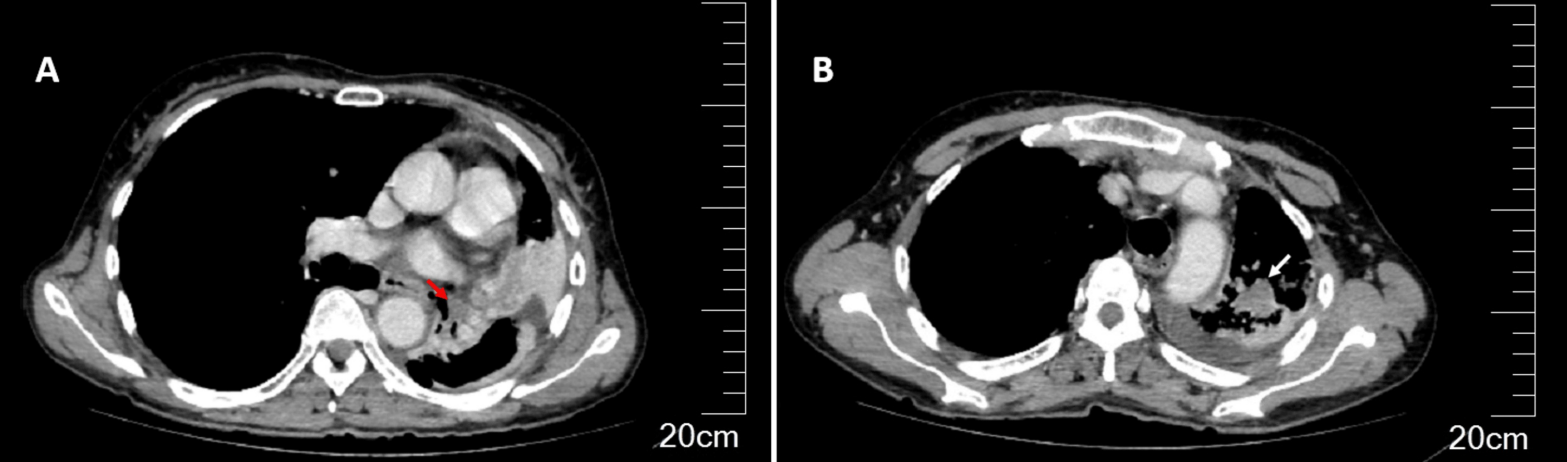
Imaging at presentation: endobronchial obstruction mimicking malignancy Axial chest CT images (a, b) demonstrating an intraluminal hypo-enhancing soft-tissue density lesion within the left lower lobar bronchus (red arrow), with a complete collapse of the left lower lobe. A separate posterior heterogeneous hypoattenuating left upper lobe parenchymal consolidation is also observed (white arrow), with a small associated pleural effusion.
